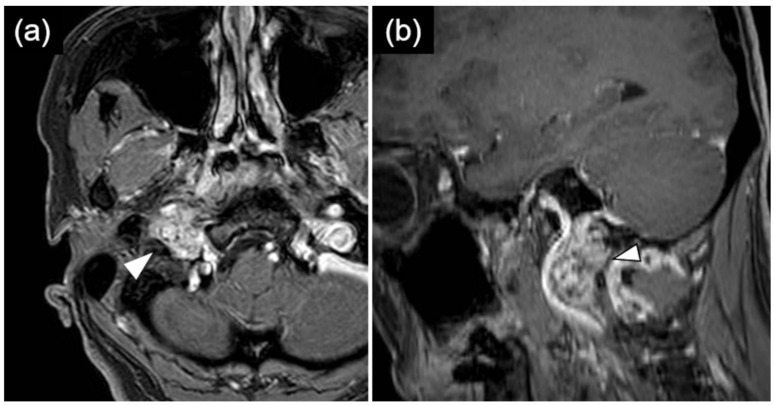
Contrast-enhanced T1-weighted magnetic resonance imaging demonstrating a 30 mm enhancing mass lesion located dorsal to the right internal carotid artery, extending extracranially and reaching the level of the first cervical vertebra (arrow head). Axial (a) and sagittal (b) views are shown.
